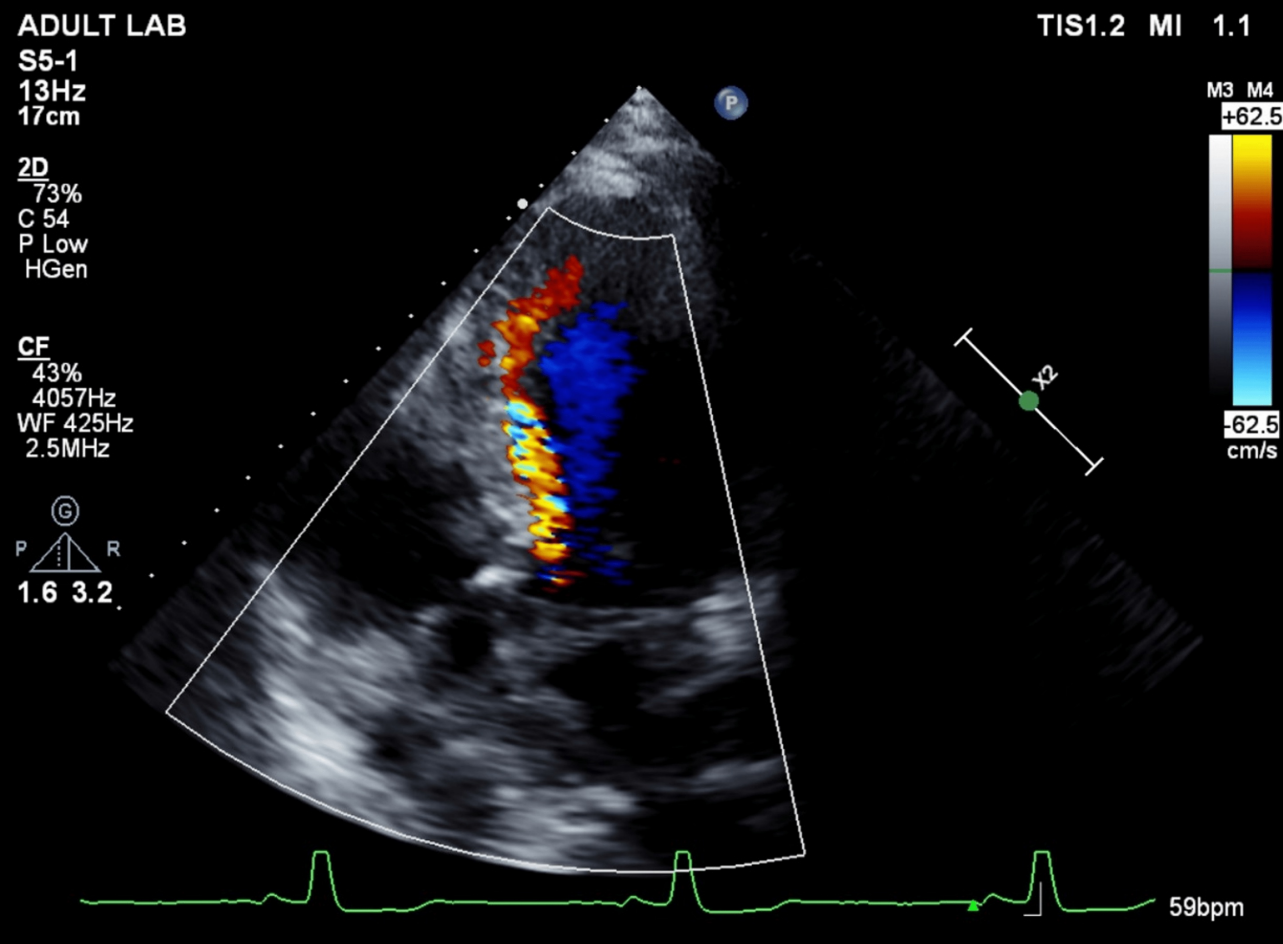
Color Doppler imaging demonstrating neo-aortic regurgitation The color Doppler imaging in an apical four-chamber view depicts a moderate-to-severe degree of neo-aortic regurgitation, which is narrow and deviated, nearly reaching the apex.
